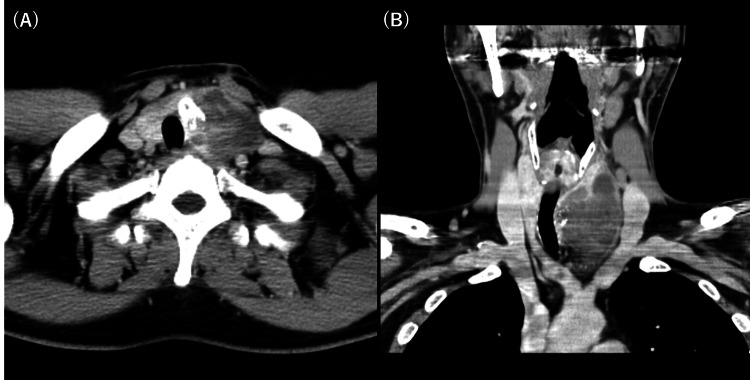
(A) Axial image; Computed tomography (CT) revealed a tumor in the left lobe of the thyroid gland, and the tumor invaded the adipose tissue around the thyroid gland on axial image. (B) Coronal image; the size of the tumor was 7 cm.
